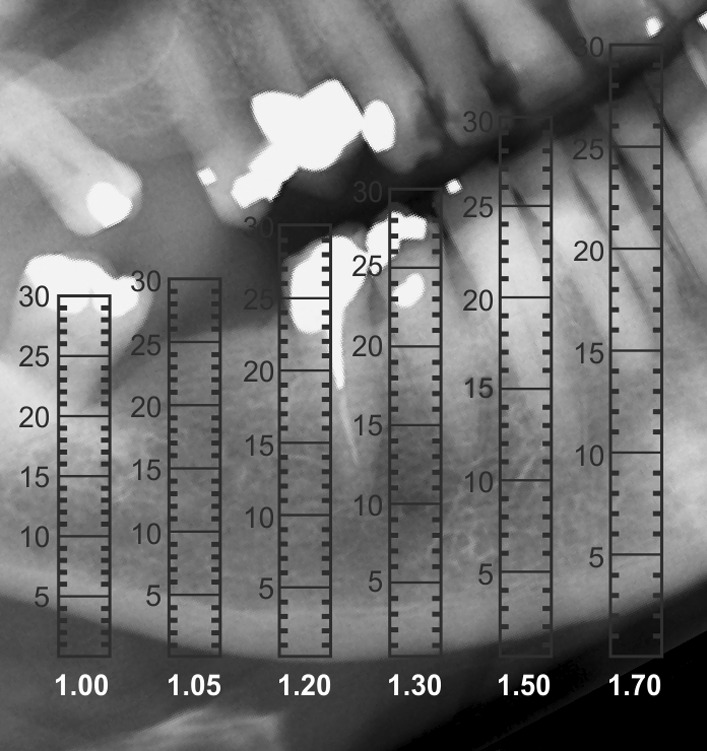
Measurement of cortical thickness on panoramic radiographs with a transparent ruler that takes into account the magnification factor (1.3)
